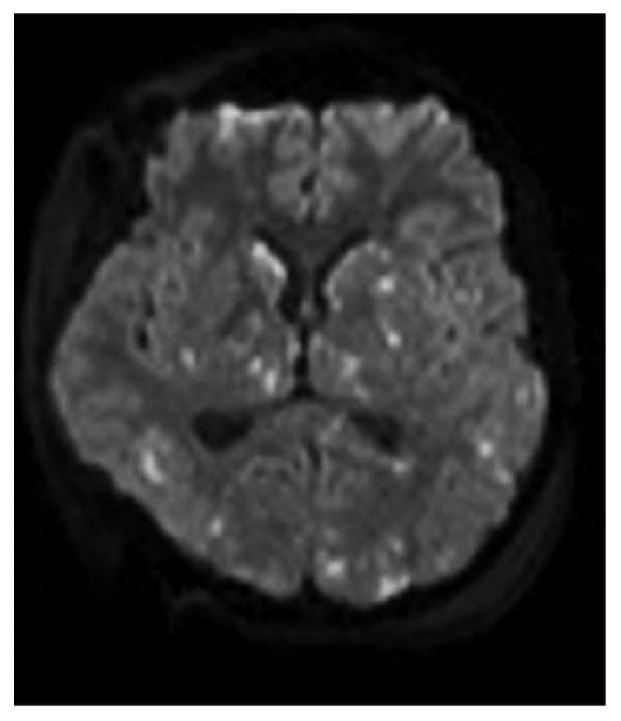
Magnetic resonance imaging, on diffusion-weighted imaging (DWI) sequences, performed 4 days after the trauma showing punctuate hyperintense foci: Starfield pattern.
